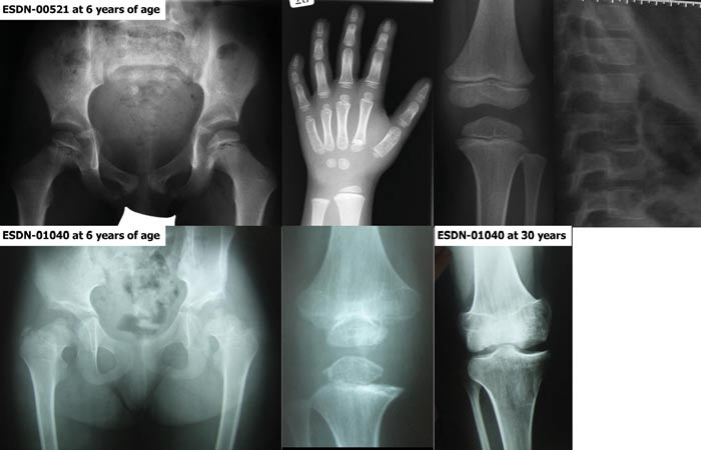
Radiographic findings in PSACH and MED patients with mutations identified in the EGF-like repeats of COMP. ESDN-00521: Radiographs taken at the age of 6 years. The proximal femoral epiphyses are small and flattened. The distal femoral and proximal tibial epiphyses are also small for age. There is no ossification yet of the proximal fibular epiphysis. The hand radiograph shows normal phalanges and metacarpals but delayed ossification of the carpal bones and epiphyses in the wrist. The spine is normal. ESDN-1040: The radiographs of pelvis and knee taken at the age of 6 years shows very small epiphyses in the hips and knees, which is reminiscent of pseudoachondroplasia. Note, also the two round translucent areas in the distal femoral metaphysis, which are often seen in patients with pseudoachondroplasia.
